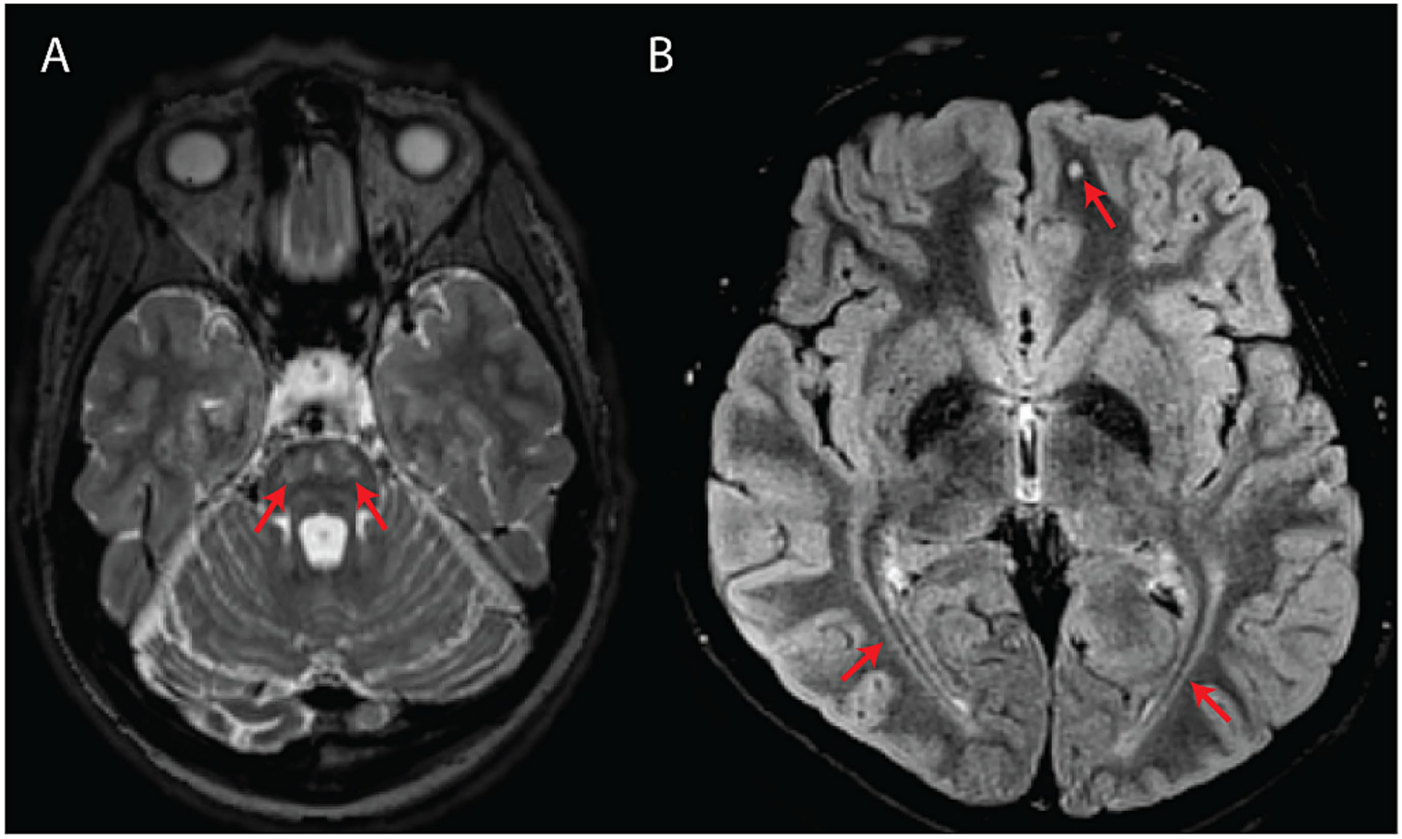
Classical MRI features associated with WFS. (A) Axial T2-weighted image showing bilateral pontine signal abnormality (subject #10). (B) Axial T2-FLAIR image showing bilateral symmetric posterior white matter abnormal signal as well as a punctate, round white matter signal abnormality in the left frontal lobe (subject #14).
